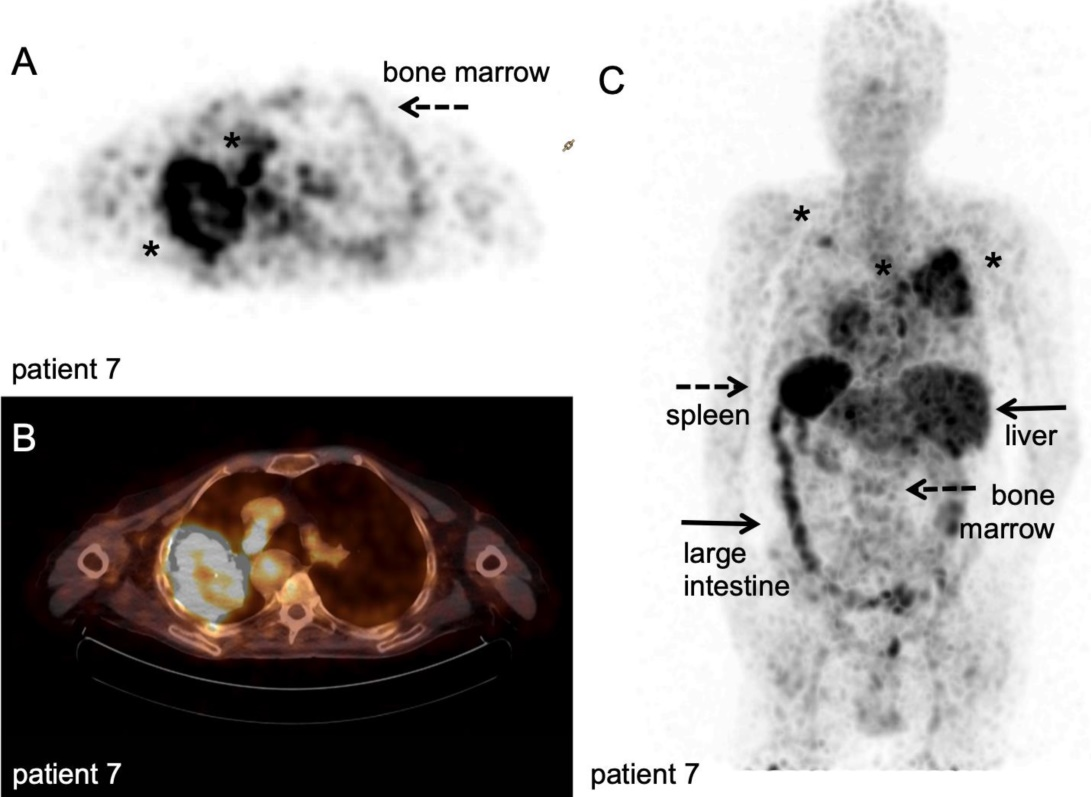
^89^Zr-nivolumab PET/CT scan. Metastatic non-small cell lung cancer patient, scanned at 162 hours after IV injection of 37 MBq [^89^Zr]Zr-DFO-nivolumab. A-B: transversal PET and fused PET/CT images; a large primary lung tumour in the right upper lobe and medial to the large mass a second lesion (asterisk). C: Maximum intensity project, posterior view; note the high uptake in the spleen (dashed arrow) and liver (filled arrows), as well as uptake in the bone marrow compartment (dashed arrow) and large intestine (filled arrow). Both lesions in the right lung were visualised (asterisk), as well as another lesion in the left upper lobe (asterisk). *With courtesy of J. de Lange*
